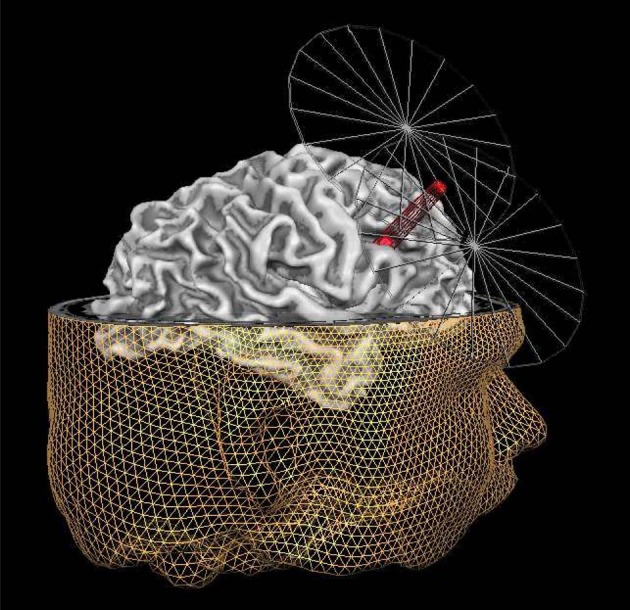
Graphic representation of the MRI neuronavigated C-TBS at the right IFS. The inferior frontal sulcus (IFS) target point (red dot under the beam of the coil) for TMS, shown on the reconstruction of the right hemisphere of one exemplary participant. The target point is placed on the posterior part of the right IFS, in particular the area just anterior to the section of the precentral sulcus and the inferior frontal sulcus. In addition to the reconstruction of the right hemisphere of this participant, also the reconstruction of the head is displayed together with a simplified visualization of the coil. The tip of the red beam from the TMS figure-8 coil indicates the site of the maximal stimulation.
